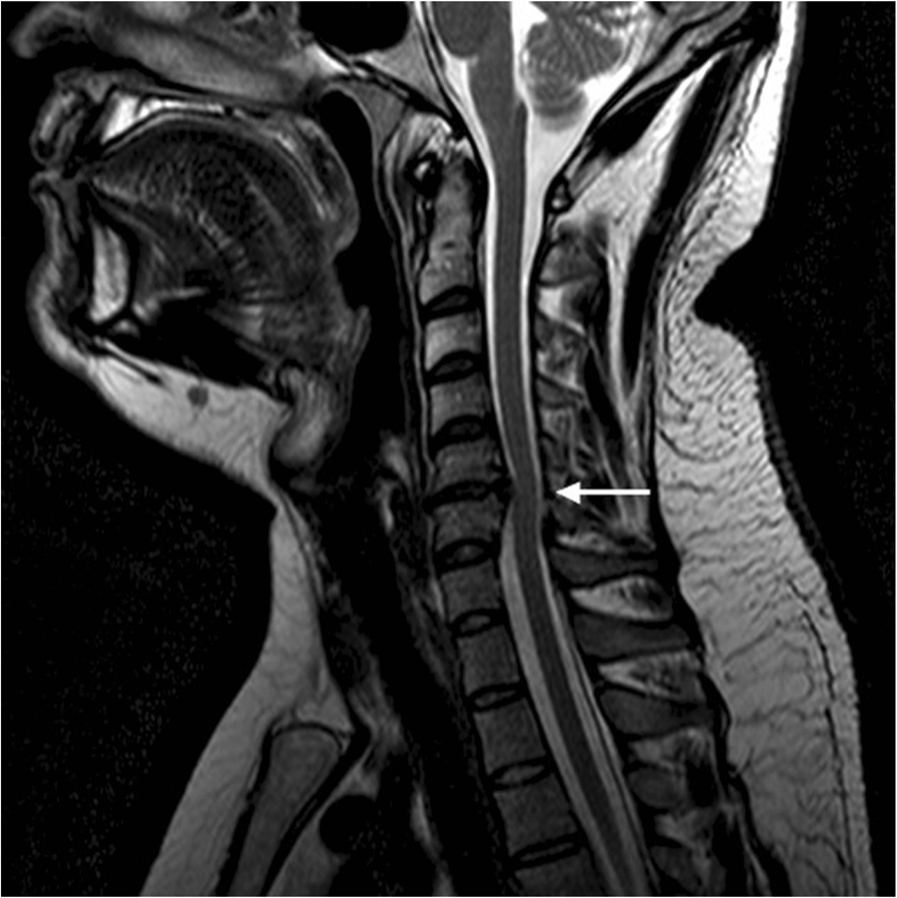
Pre-operative magnetic resonance imaging scan. The arrow on the sagittal T1-weighted cervical spine image shows the central canal is severely narrowed.
